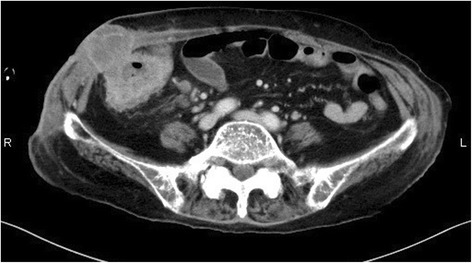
CT performed 39 days after the initial surgery demonstrating a mass that had originated from the ascending colon and had invaded the abdominal wall
